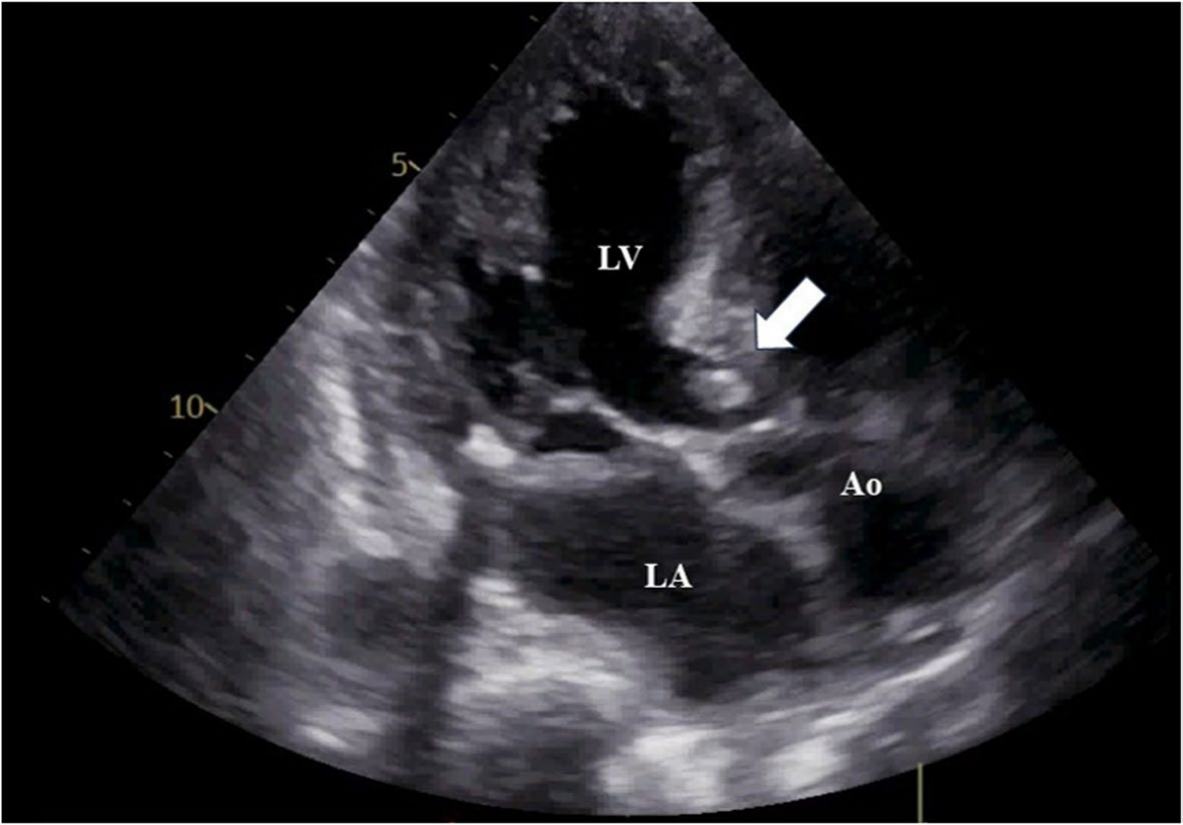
Preoperative transthoracic echocardiography shows a tumor with large mobility(arrow) in the left ventricular outflow tract. LV: left ventricle, LA: left atrium, Ao: aorta
